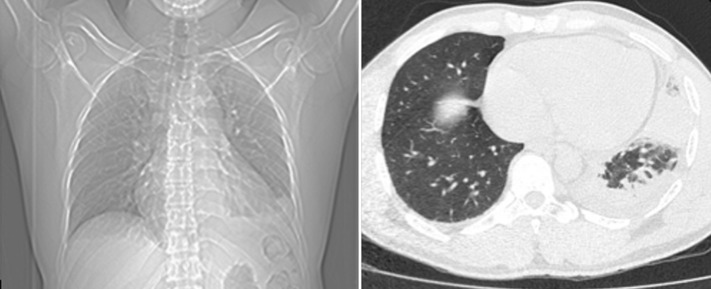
chest radiograph shows a left basal consolidation and small bilateral pleural effusions worse on the left; CT scan of the chest shows consolidation at the left base with a central area of necrosis and bilateral pleural effusions worse on the left
